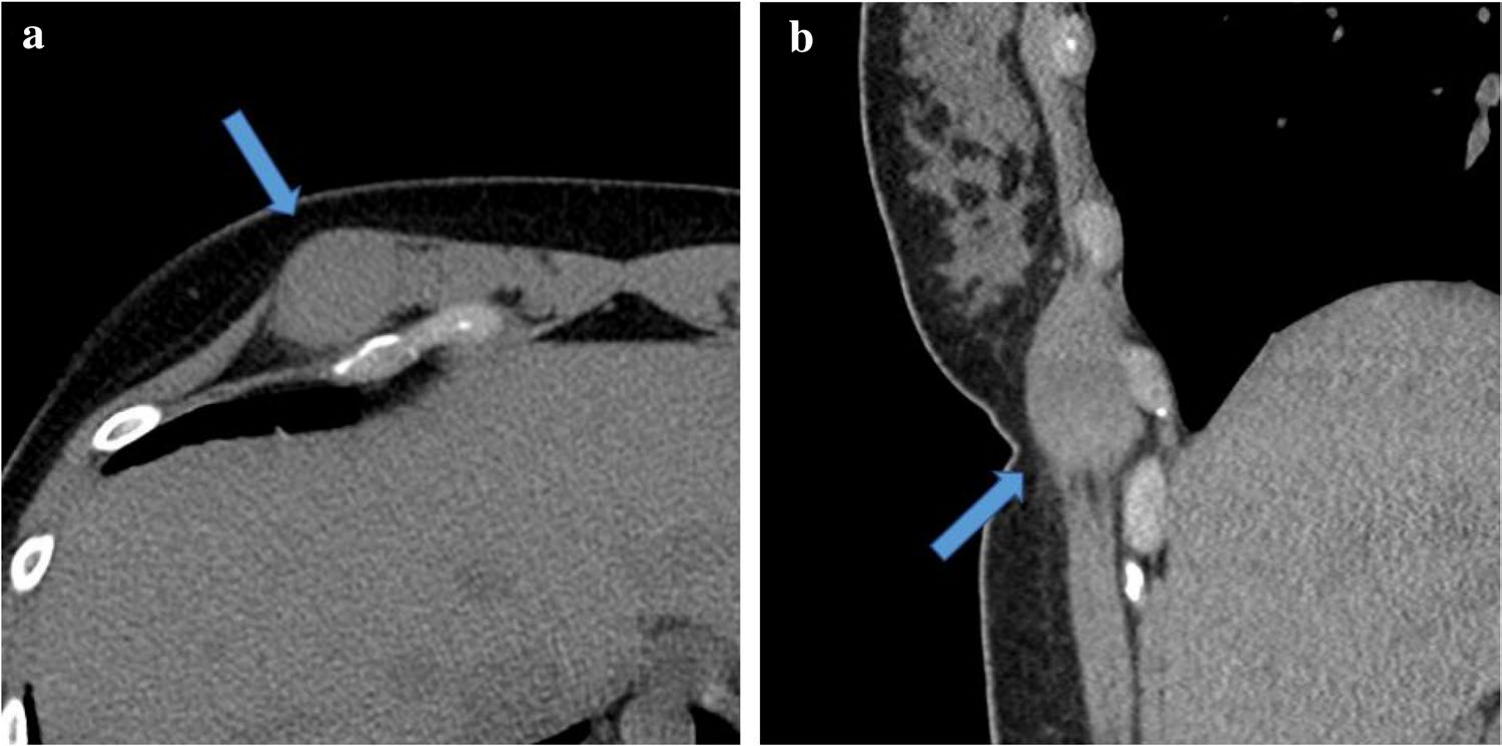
Thirty-five-year-old female with granular cell tumor of the right rectus abdominis muscle. Axial (a) and sagittal (b) CT chest redemonstrates the right upper abdominal wall mass arising from the superior right rectus abdominis muscle, without evidence of metastatic disease in the thorax (not shown). The mass is iso-attenuating to the surrounding abdominal wall musculature. There is very mild peritumoral fat stranding
